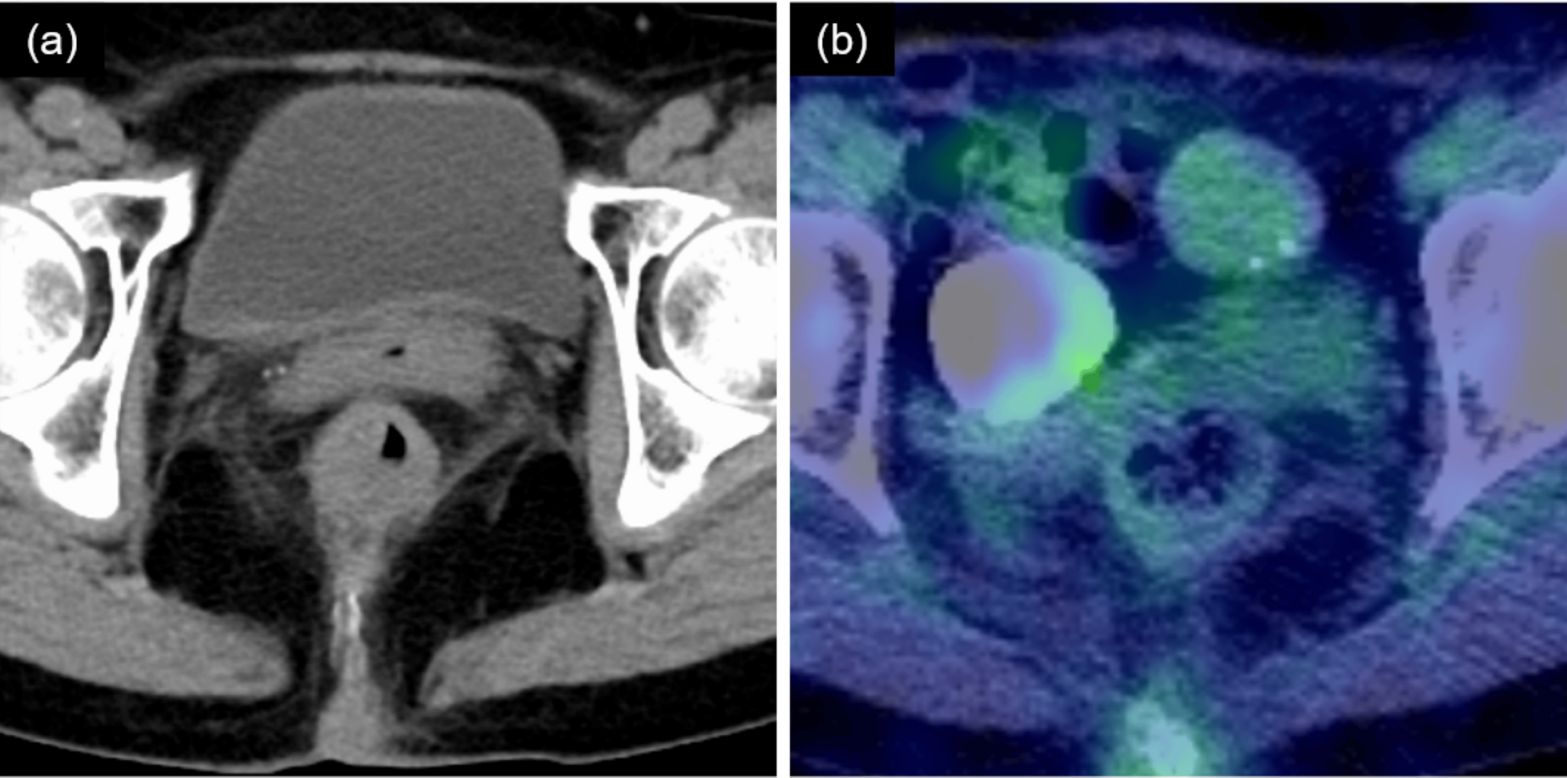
Images after radiotherapy. (a) Axial view of CT. (b) Axial view of FDG-PET/CT. SUVmax: 2.5. CT: computed tomography; FDG-PET/CT: 18F-fluorodeoxyglucose-positron emission tomography/CT; SUVmax: maximum standardized uptake value
